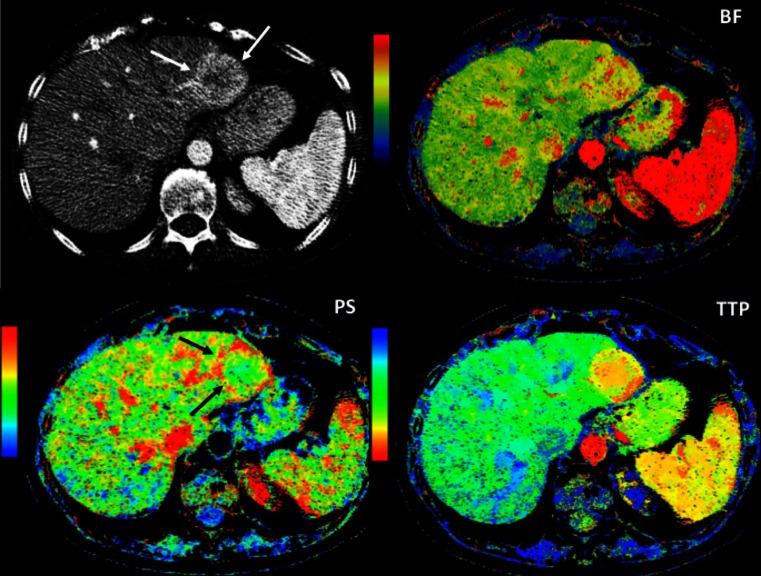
Perfusion CT images in a patient with NET liver metastases. Conventional CT image depicts a hypervascular liver metastasis in the left liver lobe (*white arrows*). Parametric maps of blood flow (*BF*), permeability (*PS*), and time to peak (*TTP*) show a different functional behavior at the periphery of the metastatic deposit (*black arrows*) compared to normal liver or the center of the metastasis, with increasing BF and PS and decreasing TTP. Perfusion CT provides additional information compared to morphologic imaging that may have prognostic value or be useful in tumor response evaluation
